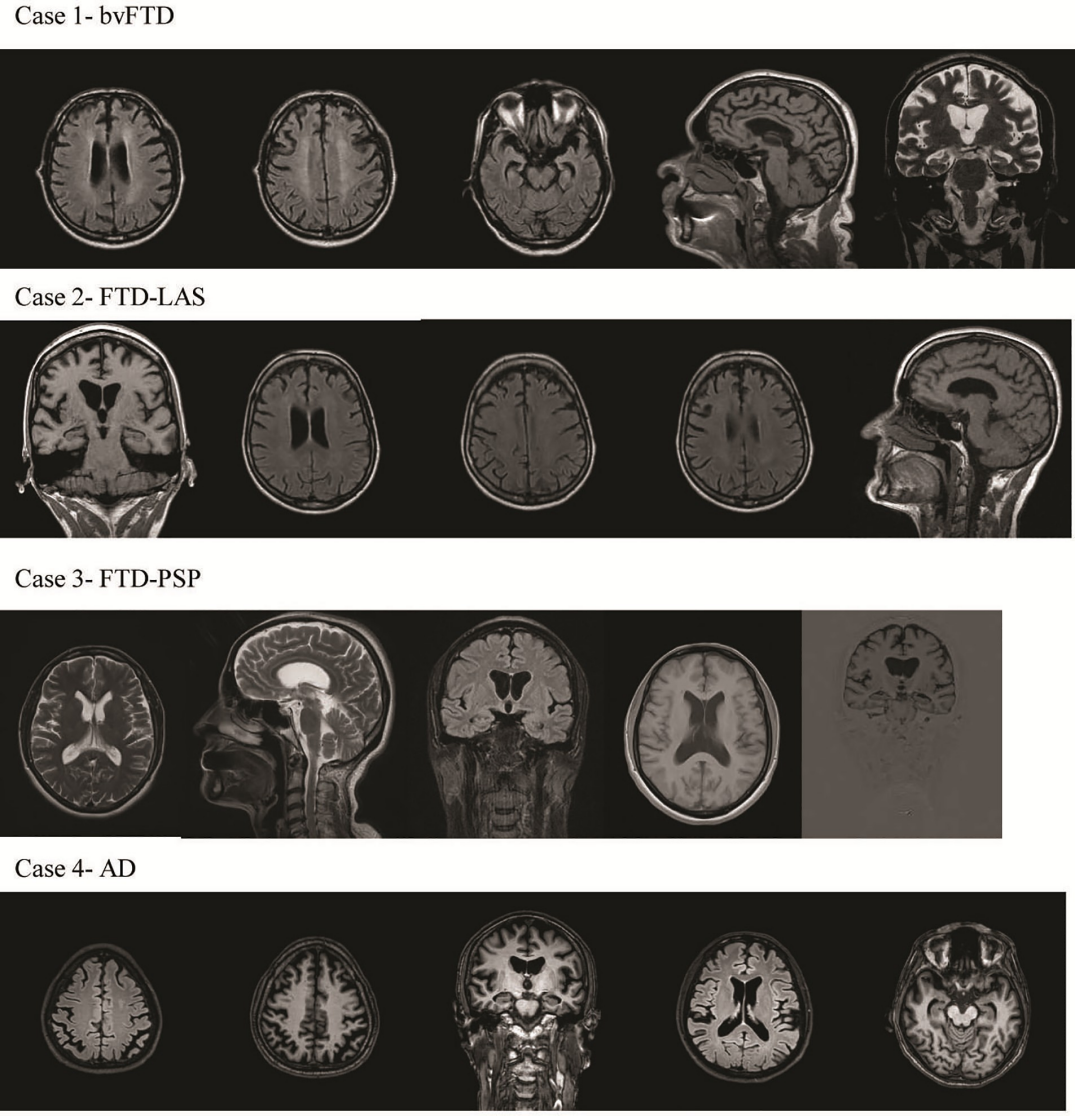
Brain MRI (T1, T2 and Flair) of *C9orf72* expansions in the Bulgarian cohort (Case 1, 2, 3, 4). Case 1—Bilateral frontal and temporal atrophy with very mild asymmetry that was more pronounced in the left hemisphere and hippocampal atrophy; Case 2—Mild frontal atrophy with very mild asymmetry that was more pronounced in the left frontal lobe and very mild hippocampal atrophy; Case 3—Mild hippocampal atrophy; Case 4—Generalized cortical atrophy, including posterior atrophy and bilateral hippocampal atrophy.
